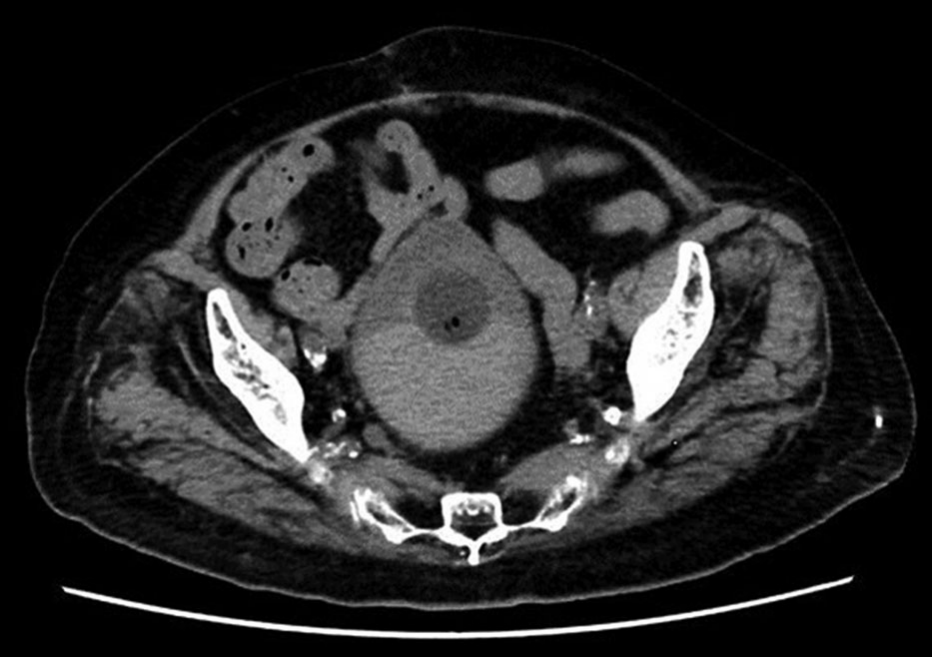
Computed tomography scan showing blood clots in the bladder, but no ascites.
